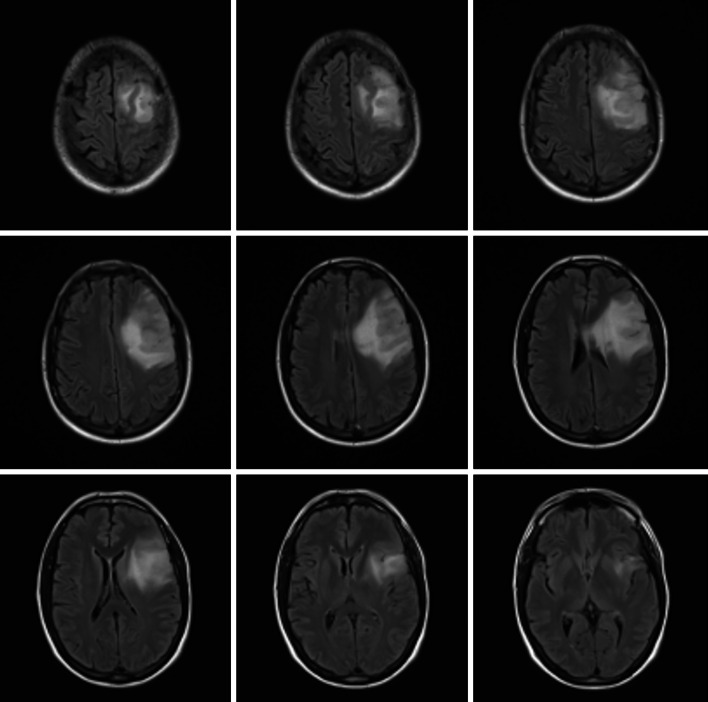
How would you treat this patient? A 52 year old male, right hand dominant, presents with simple partial seizures with motorized aphasia. Neurologically intact. MRI FLAIR sequence is shown. There was NO enhancement with gadolinium
